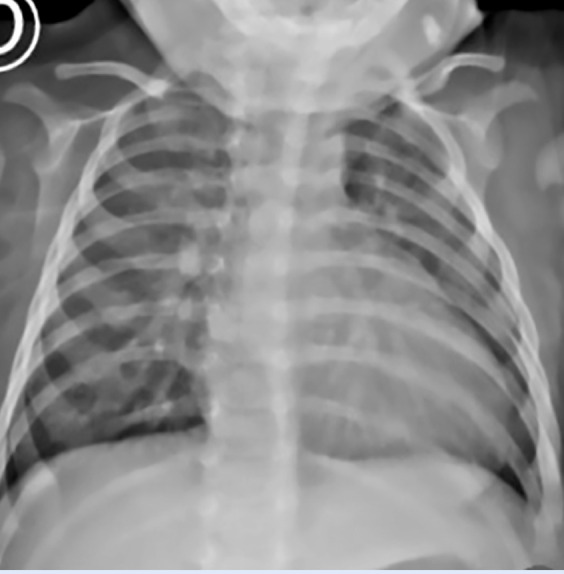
Chest X-ray on patient admission.
